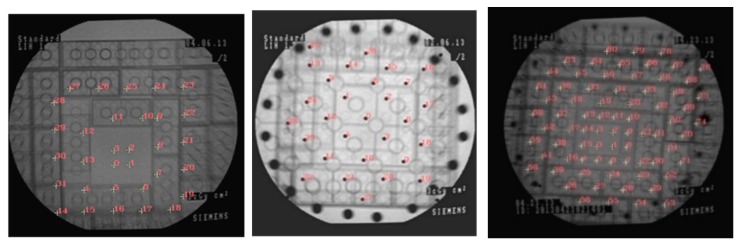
2D point localization in the X-ray images.
